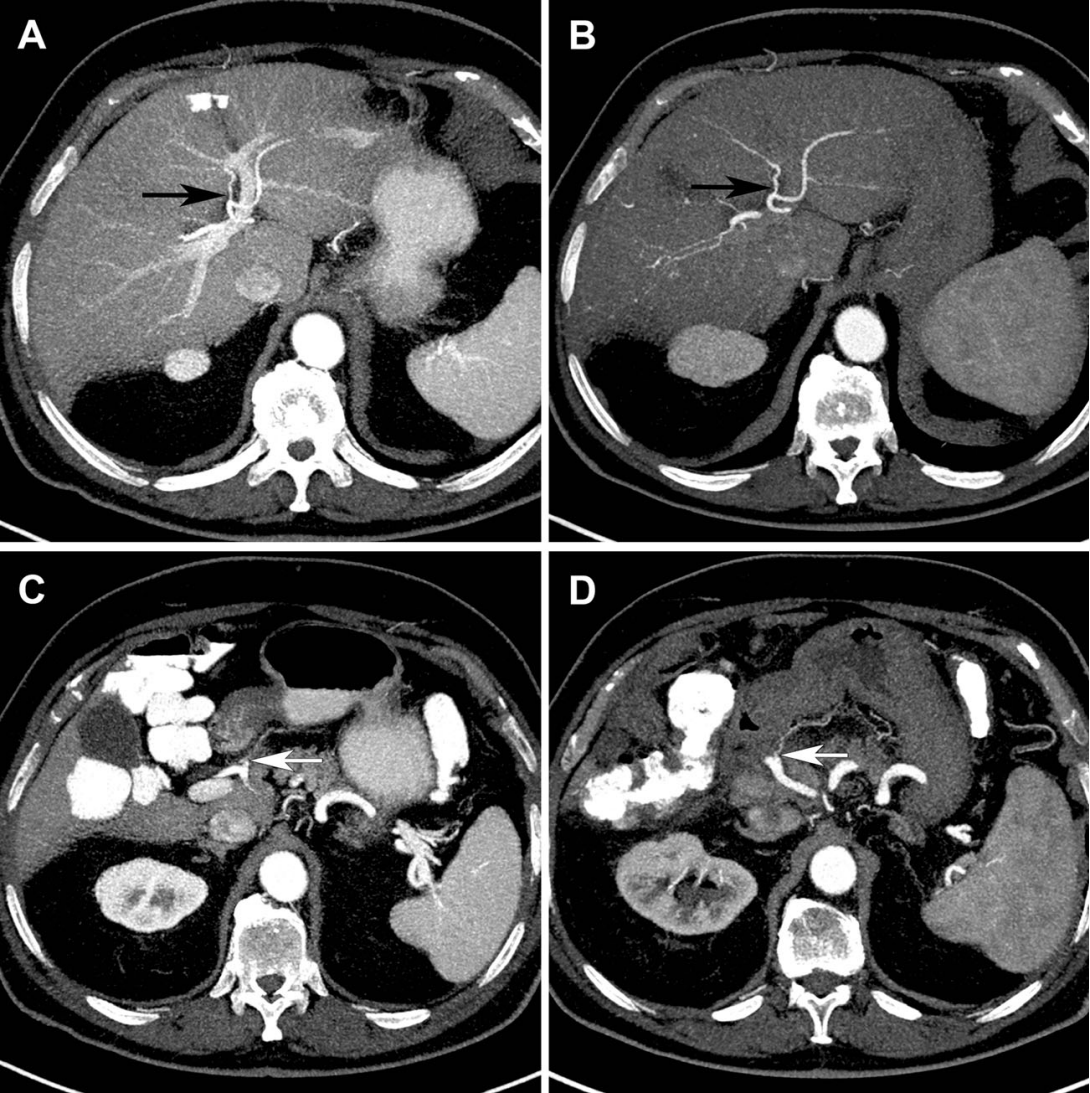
a-d Comparison of maximum intensity projections of liver CT images with the standard (a, c) and the adjusted arterial phase protocol (b, d) in the same patient, acquired on the same scanner. Note that the adjusted protocol is easier to evaluate due to an increased contrast-to-noise ratio for the hepatic artery relative to the portal vein, but the origins of the A4 (a, b, black arrow) and the right gastric artery (c, d, white arrow) are nonetheless visible in both protocols
